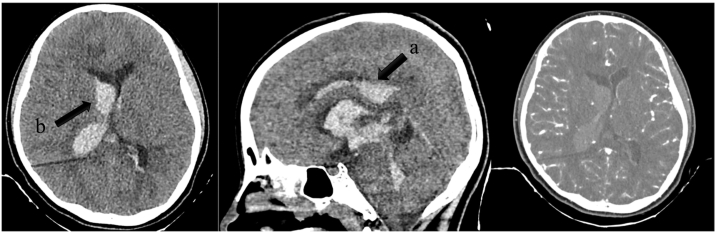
Head CT of the second case revealed the presence of a hyperdense lesion on the right parietal white matter 2.2 × 6.8 × 2.6 cm in size with a continuation to and filling the right and left lateral, third, and fourth ventricles (a), along with dilation indicating intracerebral & intraventricular hematoma with non-communicating hydrocephalus (b). CTA did not reveal any apparent vascular malformation.
